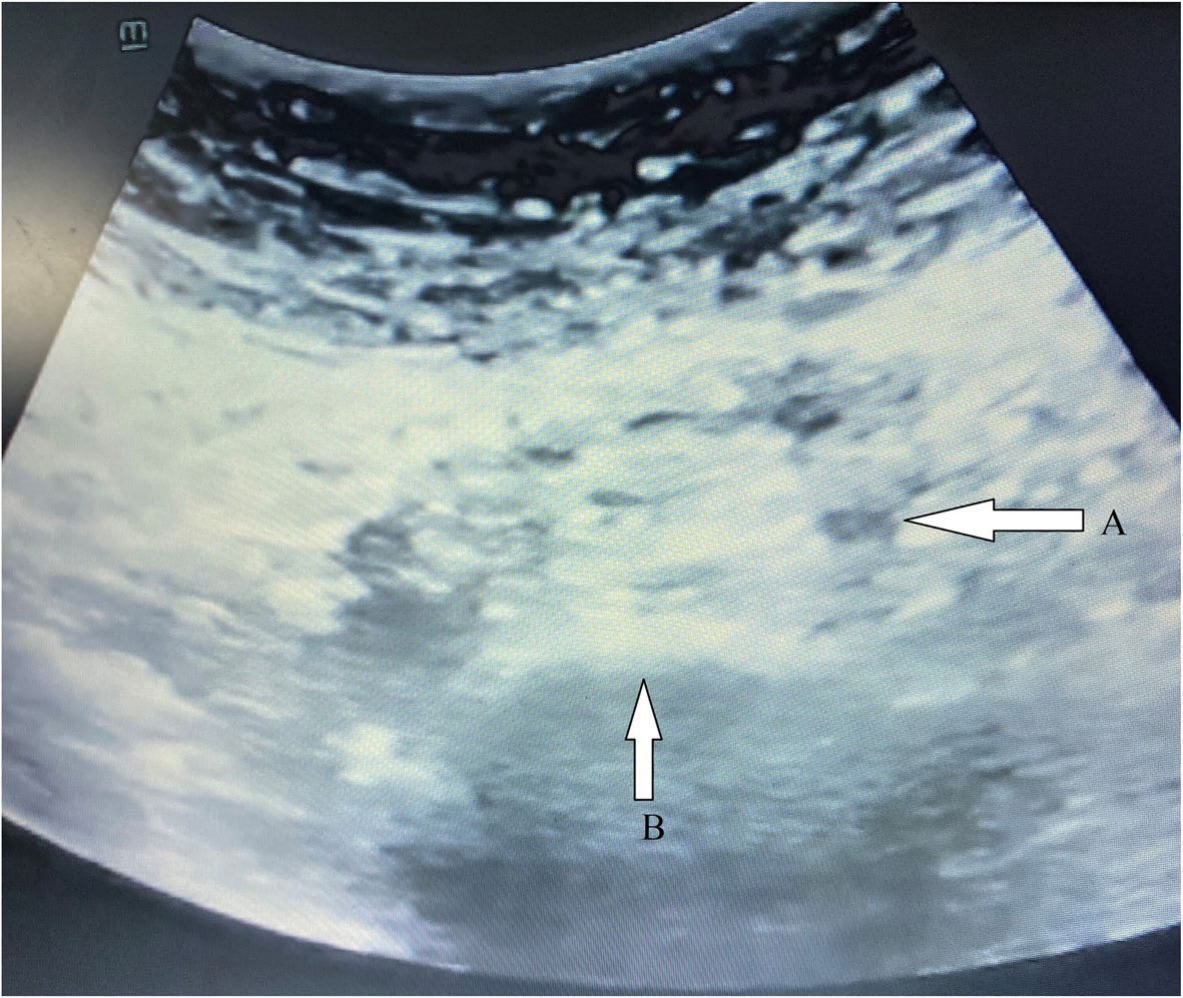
Ultrasound view showed the common iliac vessels (A) and the body of the fifth lumber vertebra (B)
